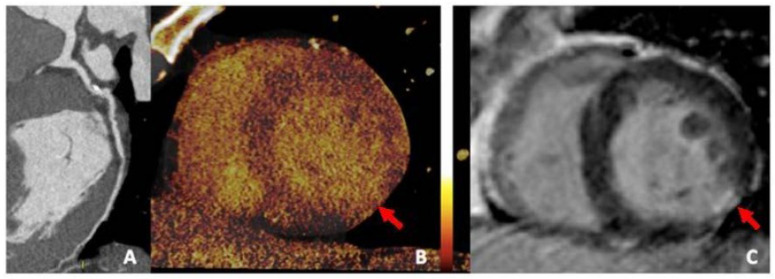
DECT of the left circumflex coronary artery. (A) Image depicting severe proximal stenosis due to the presence of calcified plaque inside the left circumflex coronary artery. (B) Iodine perfusion mapping demonstrating a defect in the inferolateral wall (arrow) corresponding to a prior myocardial scar (arrow). (C) Necrosis (arrow) caused by persistent myocardial ischemia of the inferolateral wall. DECT = dual-energy CT. Source: Dell’Aversana, S.; Ascione, R.; De Giorgi, M.; De Lucia, D. R.; Cuocolo, R.; Boccalatte, M.; Sibilio, G.; Napolitano, G.; Muscogiuri, G.; Sironi, S.; et al. Dual-energy CT of the heart: a review. *J. Imaging* 2022, *8*, 236.
